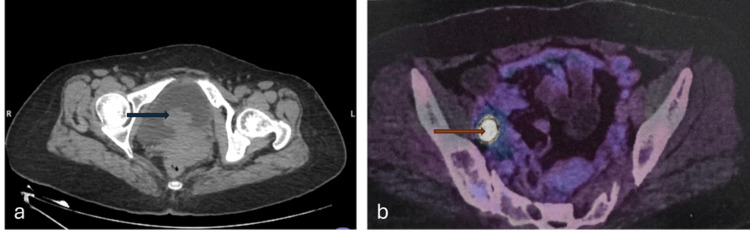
CECT and DOTANOC PET/CT findings (A) CECT scan showing a broad-based polypoid macrolobulated arterial-enhancing intraluminal mass lesion, measuring 3.6×2.9×2.2 cm (CC×AP×TS), epicentered in the postero-inferior wall of the urinary bladder (blue arrow). (B) DOTANOC PET/CT showing the appearance of ^68^Ga-DOTANOC avid right external iliac node (orange arrow), measuring ~ 13×10 mm (SUV max ~71). CECT: contrast-enhanced computed tomography; CC: craniocaudal; AP: anteroposterior; TS: transverse section; PET: positron emission tomography; CT: computed tomography; SUV: standardized uptake value
